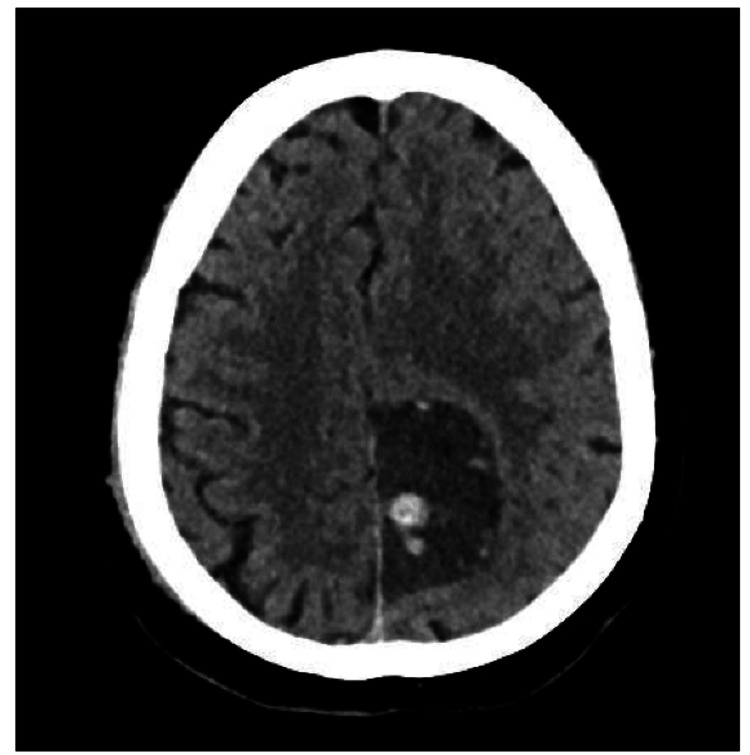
A cross-sectional CT view of the brain shows a well-defined hypodense lesion in the left parietal region, adjacent to the falx cerebri. This lesion is associated with multiple rounded, hyperdense components, most likely representing hemorrhage. There is a mass effect on the adjacent structures, although no midline shift is observed.
